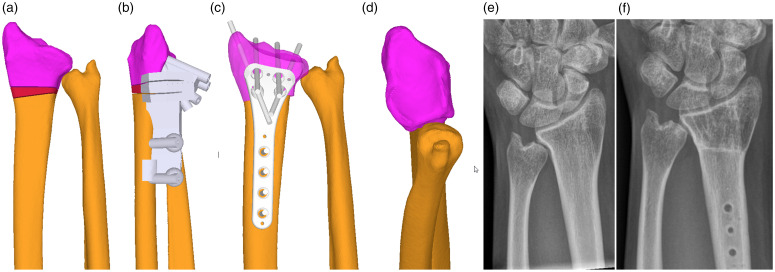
Congenital ulnar-minus variance with preoperative computer-assisted, three-dimensional planning of the radius osteotomy and distal radioulnar joint (DRUJ) reconstruction. (a) Three-dimensional bone model of pathologic DRUJ, proximal radius and ulna (orange), bone wedge (red), distal radius (pink), (b) drill and saw guide (grey), (c) distal radius plate (grey), (d) articular view of the reconstructed DRUJ, (e) preoperative anterior–posterior radiographs of the wrist, (f) postoperative anterior–posterior radiographs of the wrist.
